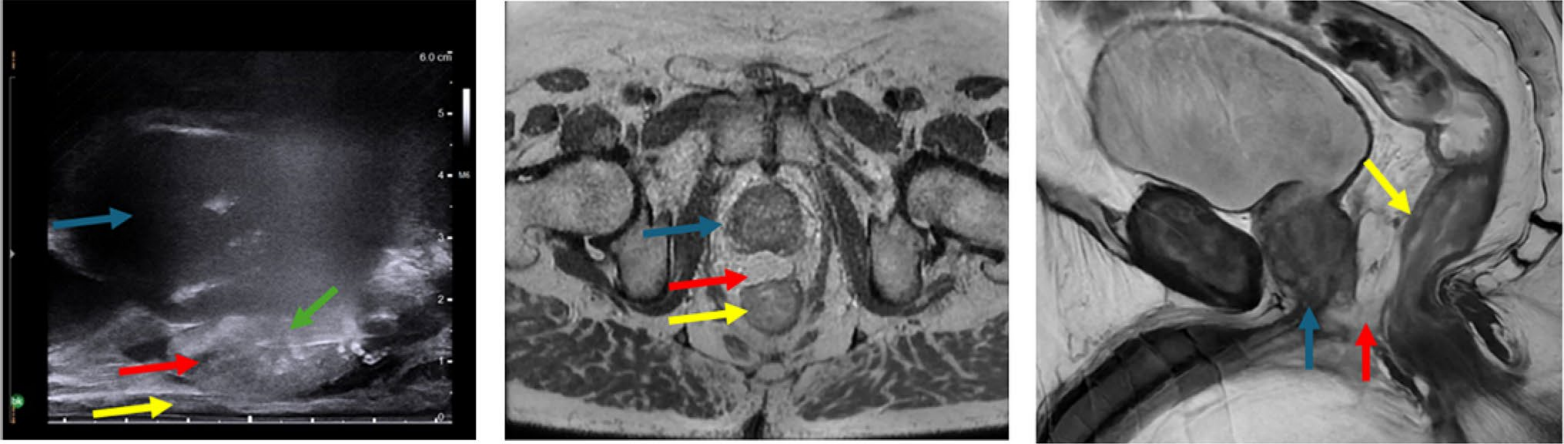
Longitudinal ultrasound image after injection of hydrogel spacer (red arrow) in the perirectal space between the prostate (blue arrow) and anterior rectal wall (yellow arrow) via an 18G needle (green arrow). Subsequent axial and sagittal T2 MRI demonstrates appropriate position of hydrogel spacer (red arrow) between the prostate (blue arrow) and rectum (yellow arrow)
